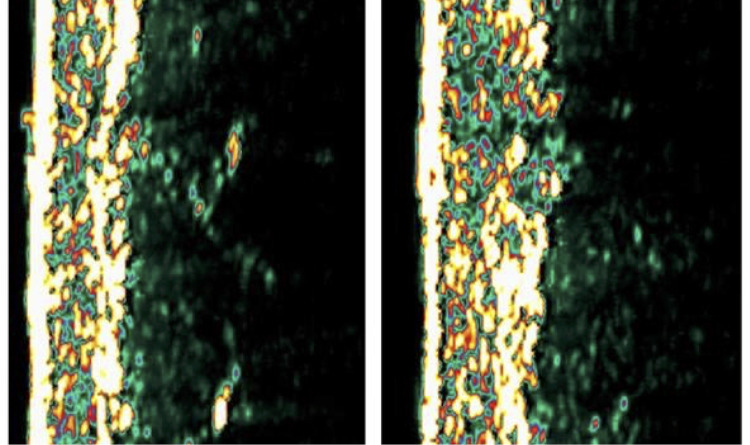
Ultrasound imaging of the upper arm. Left: pre-treatment baseline. Right: post-treatment assessment demonstrating changes in dermal structure following PLLA administration.
